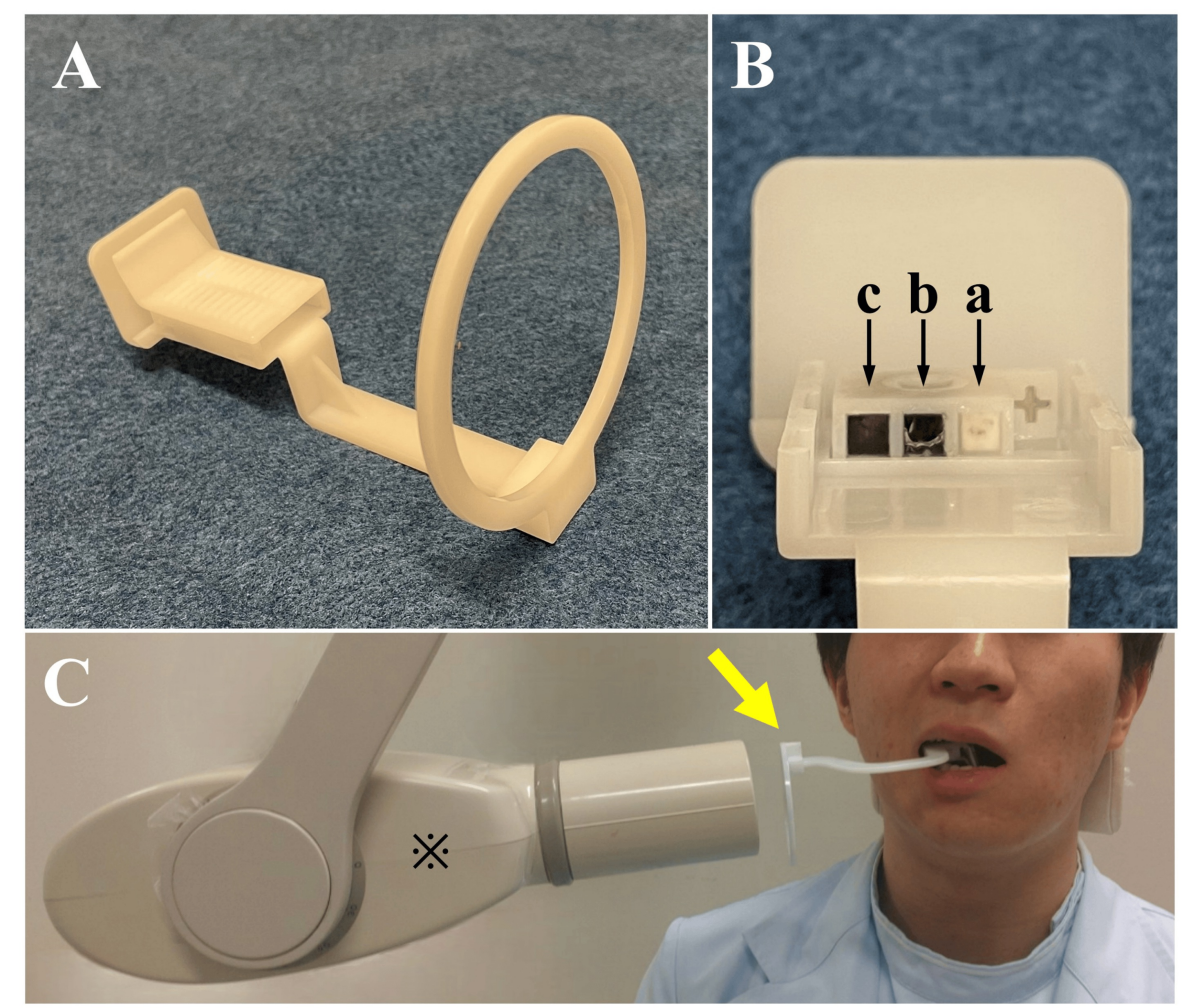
A specialized indicator A specialized indicator (A) was employed during imaging and contains calcium carbonate reference standards (B) for alveolar bone mineral density (al-BMD) (20%: 500 mg/cm³ (a), 60%: 1000 mg/cm³ (b), and 100%: 1500 mg/cm³ (c)). (C) shows intraoral radiography (※: X-ray tubehead) of the mandibular premolar region performed using a specialized indicator (yellow arrow).
